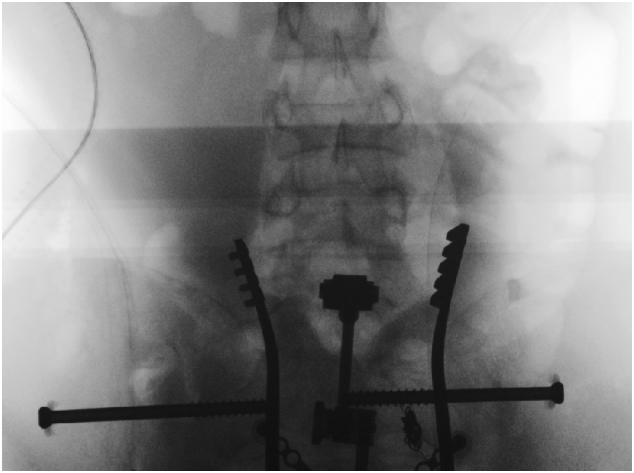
intraoperative radiograph shows fixation of the sacroilliac joint with two percutanous cannulated screws.
